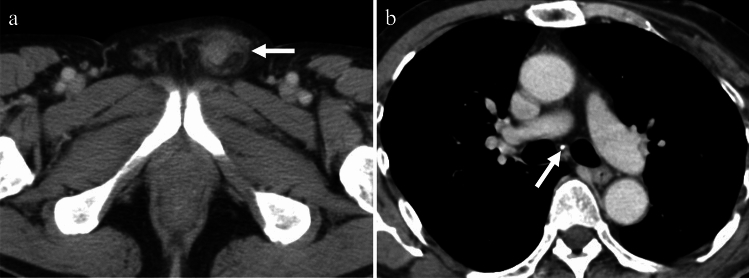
Man in his 60 s with tuberculous vasitis presenting with a left groin mass. a Contrast-enhanced CT showing swelling in the left inguinal canal (arrow) with increased peripheral fat density. b On chest CT, calcification corresponding to the mediastinal lymph nodes (arrow) is present, suggesting tuberculosis
